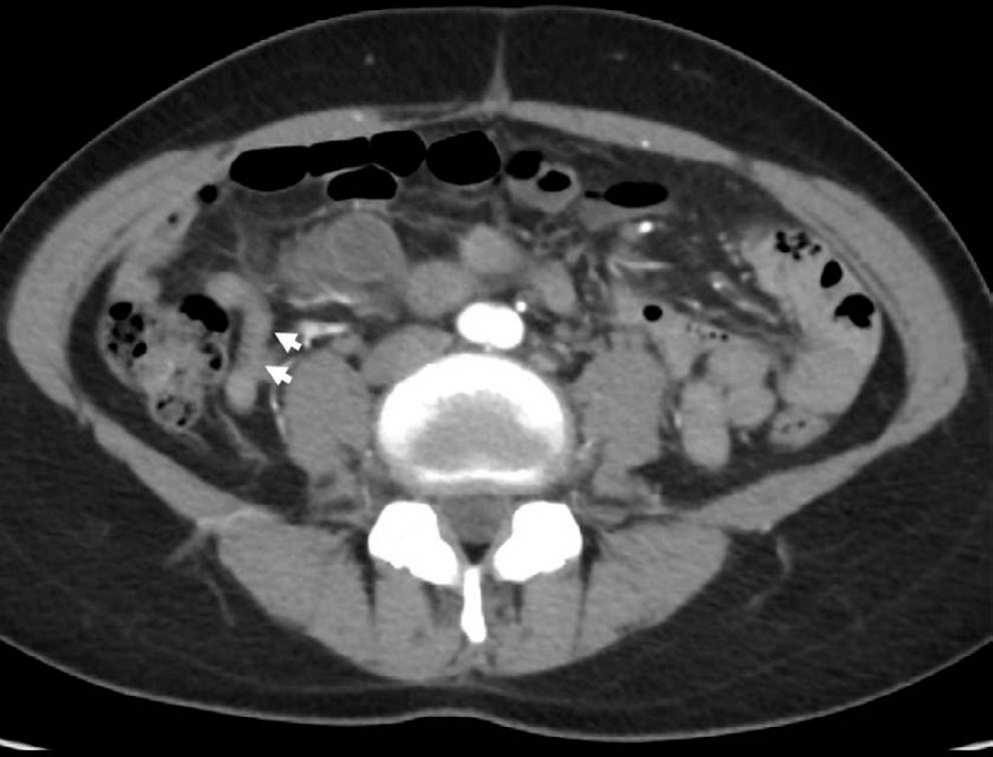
Appendix was normal based on computed tomography (0.6 × 5.5 cm, white arrows)
